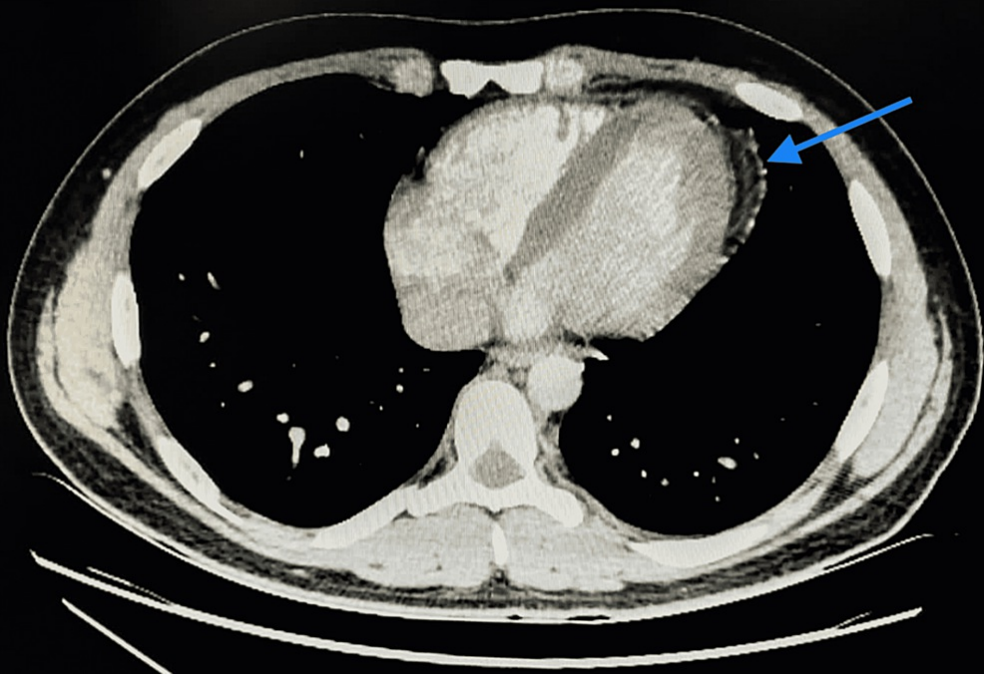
CT angiocoronal view showing pericardial fat stranding (blue arrow).
